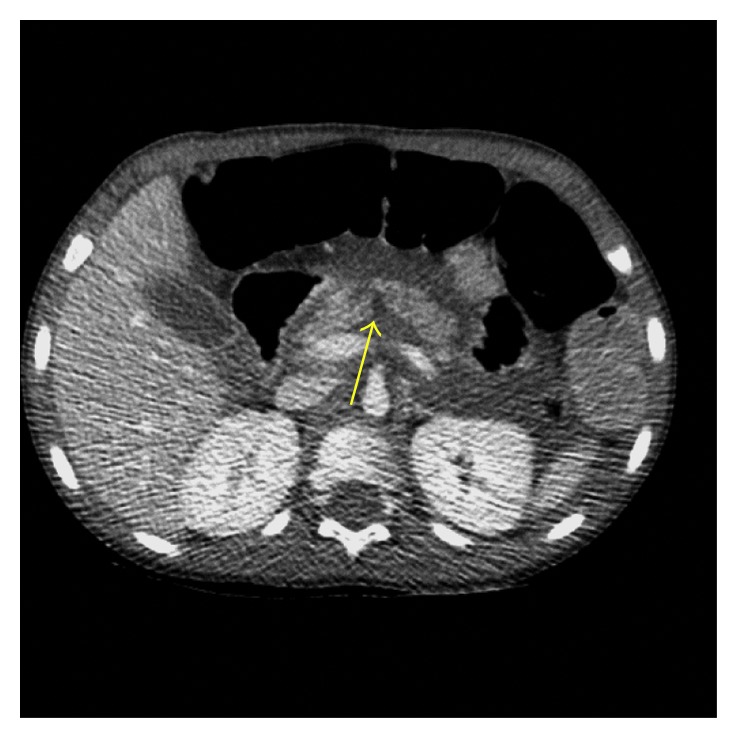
CT of abdomen showing linear low attenuation within the pancreatic body.
